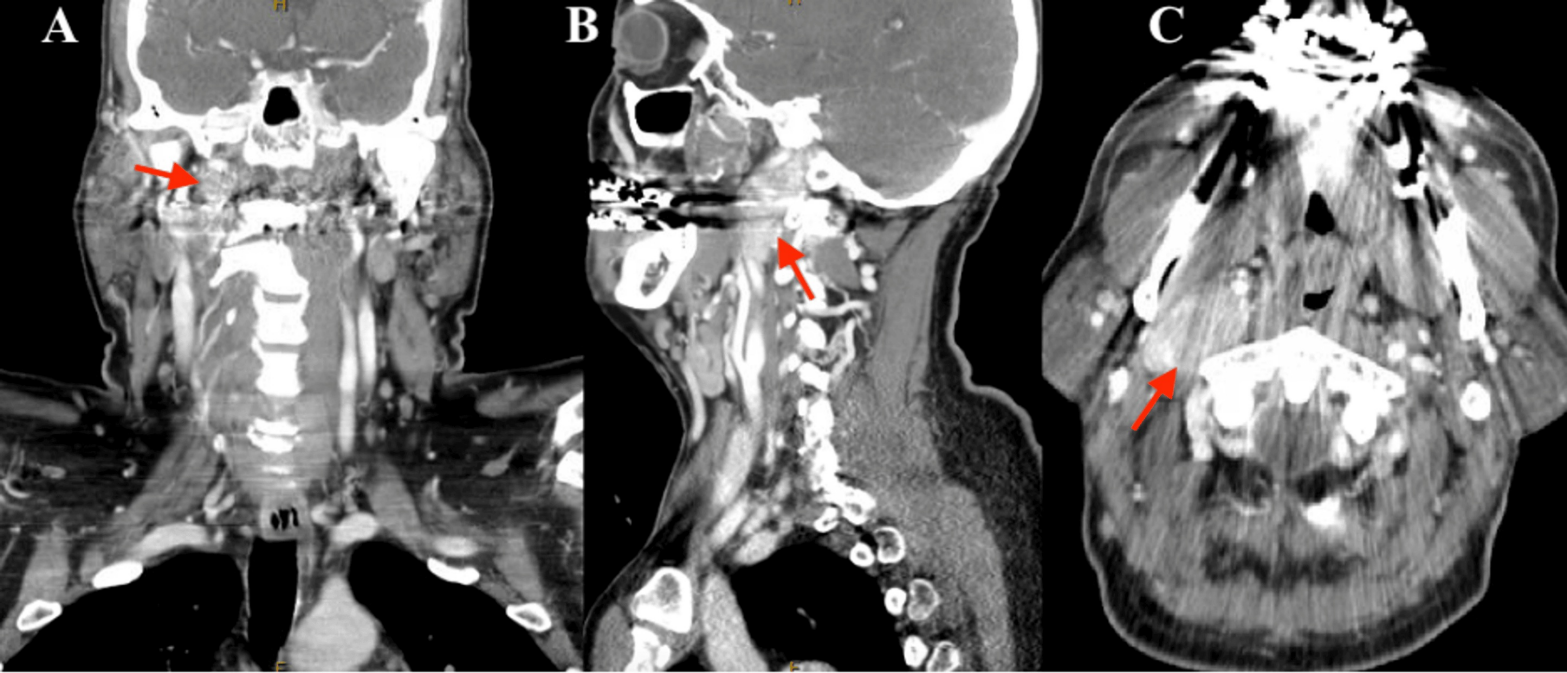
Computed tomography showing an enhancing mass (red arrows) along the right skull base with extension into the jugular foramen and hypoglossal canal. The internal carotid artery is displaced anteromedially. A) Coronal. B) Sagittal. C) Axial.
